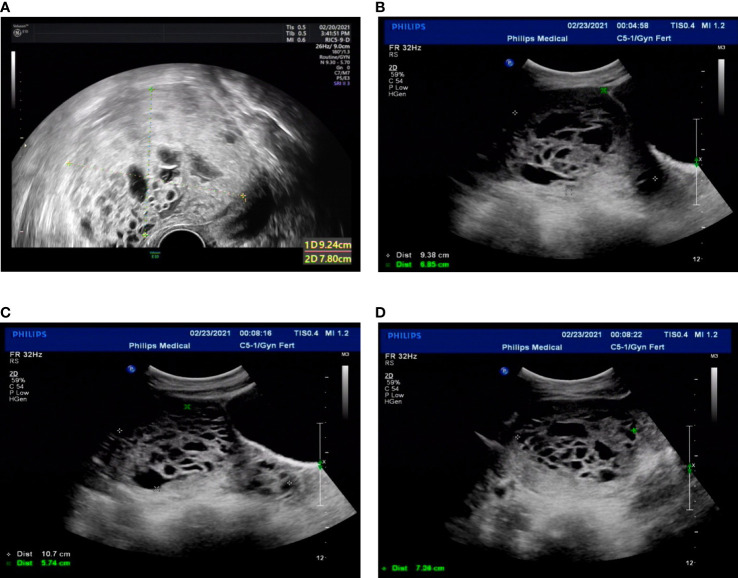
Transvaginal ultrasound. (A) Twenty-one days after the second molar evacuation. (B-D) The ultrasonographic image when the uterus perforation. The uterus was 9.4 × 9.7 × 6.9 cm, an uneven echo area of 10.7 × 7.1 × 5.7 cm was observed in uterine cavity and cervical canal, protruding left anterior wall, local muscle layer was unclear.
